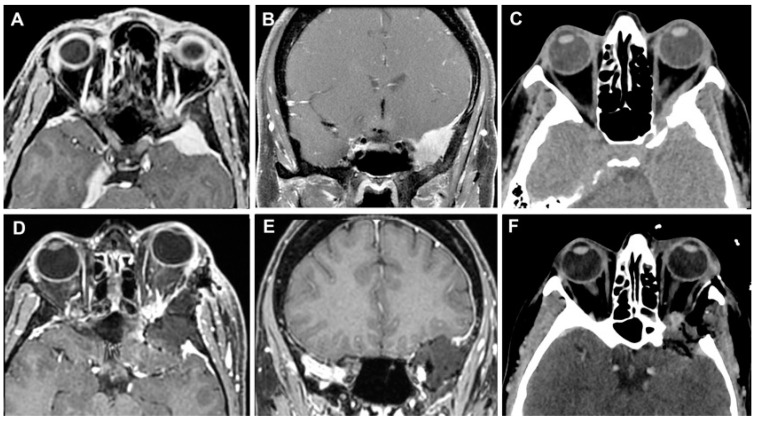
MR images (A,B) and on axial CT scan (C) of a sphenoid wing meningioma. No signs of recurrence were identified in the post-operative MRI (D,E) and CT scan (F).
